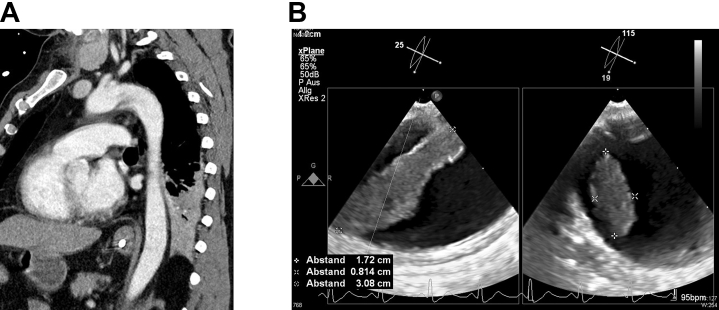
Intravascular Mass in the Aortic Arch (A) Computed tomography scan. (B) Transesophageal echocardiography.
